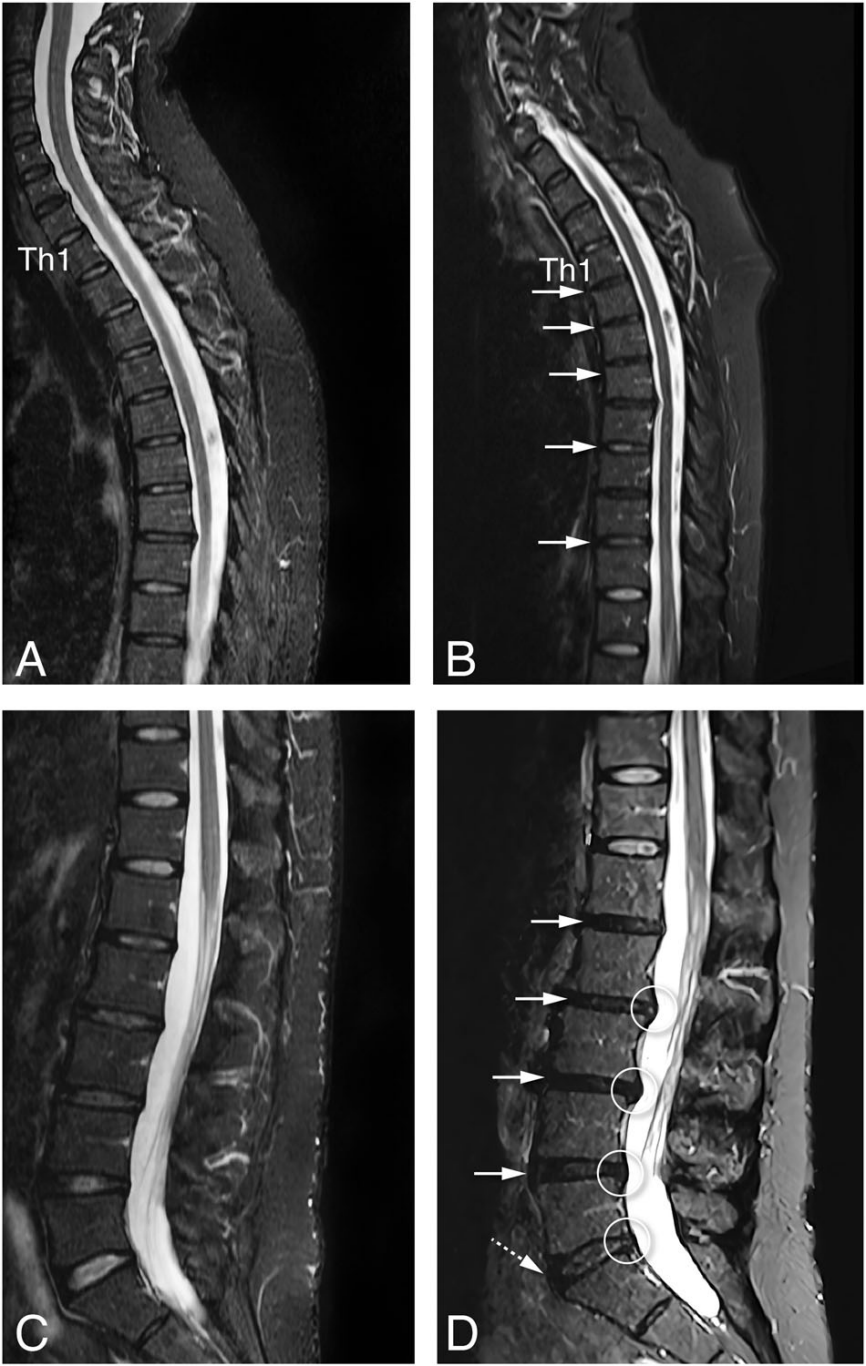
Example of a patient with a progression of DLs in the cervical, thoracic and lumbar spines on MRI over 10Y. Sagittal STIR images at baseline and 10-year follow-up of the cervicothoracic (A, B) and lumbar (C, D) spine. Decrease in height of the intervertebral disc spaces at multiple levels with a decrease of the signal of the discs Th1–Th4, Th5–Th6, and Th7–Th8 from grade II (normal discs) to grade IV (degenerated discs) (A, B). Progressive degenerative discs at all lumbar levels (white arrow) with the development of protrusion (circles) (C, D). L5-S1 (dashed arrow) progresses from grade II (normal disc) to grade III (degenerated disc)
